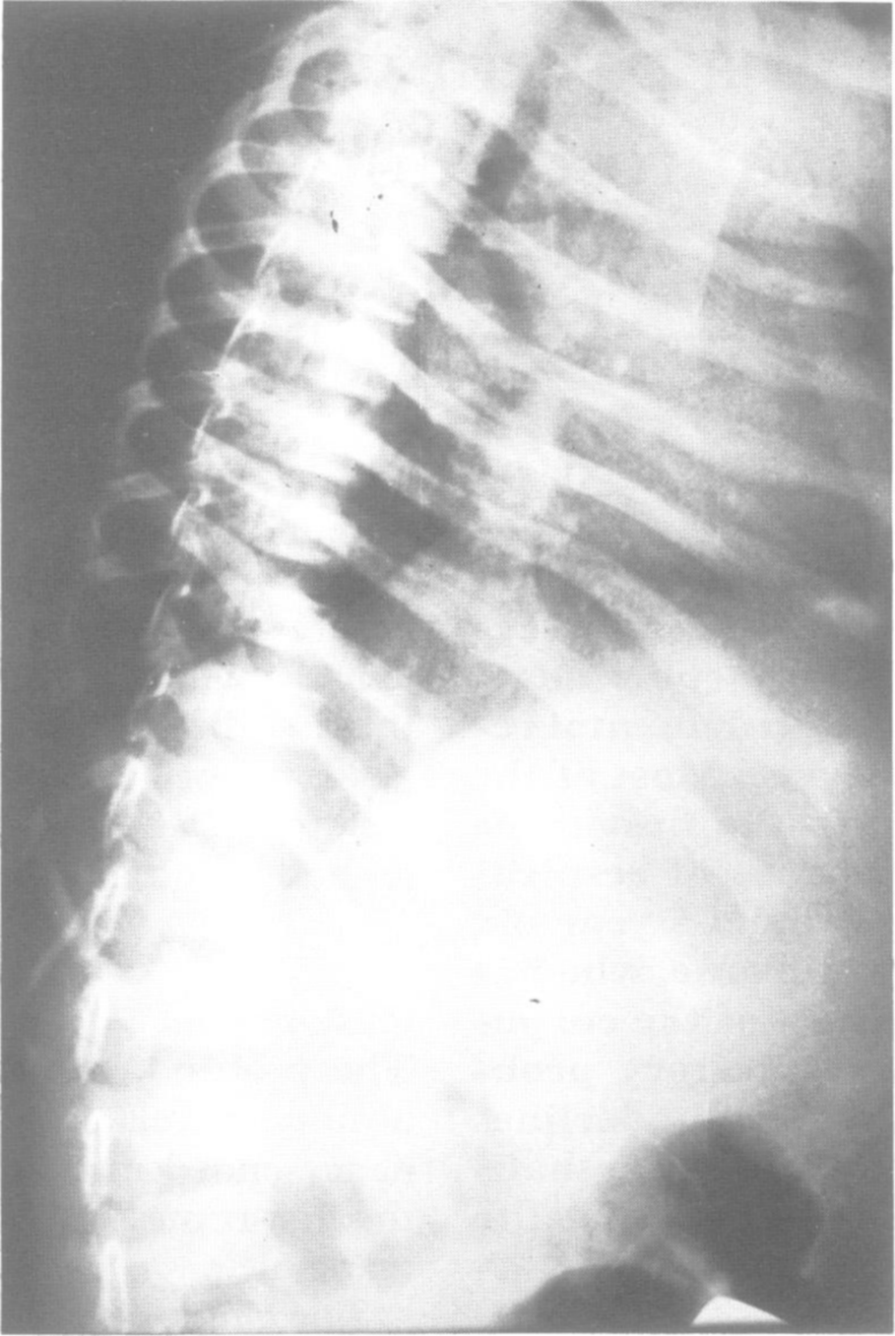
An X-ray showing thinned ribs and dysmorphic lumbar vertebrae in a 4-week old male with MSS [4].
